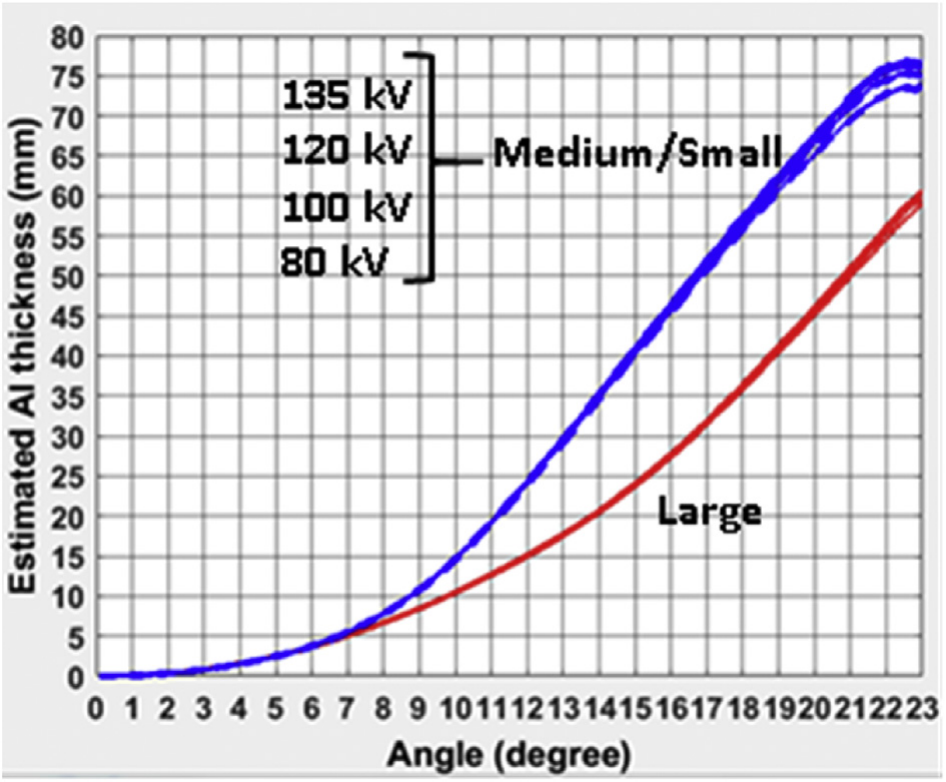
# Data of CT bow tie filter profiles from three modern CT scanners
